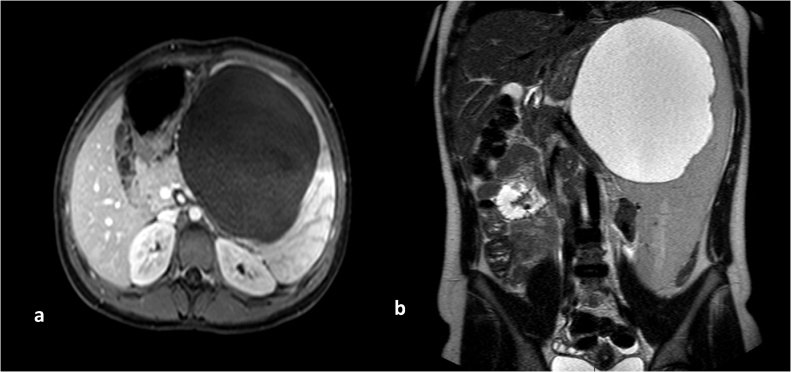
Axial T1 post contrast MR image shows a non-enhancing cystic lesion (3a), Coronal T2 image shows a hyper-intense fluid signal intensity splenic lesion (3b).
